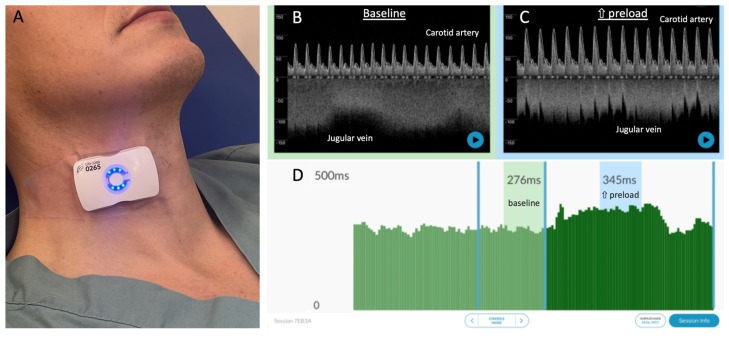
The wearable Doppler system and its graphical user interface. (A) The wireless, wearable Doppler transducer adhered to the neck of a healthy subject. (B) Representative resting baseline data from a healthy subject with common carotid and internal jugular spectrograms. (C) Representative data during increased preload showing carotid and jugular spectrograms; note the change in carotid and jugular morphologies consistent with increased preload and ccFT. (D) Per-beat ccFT calculations showing windows at baseline (average ccFT 276 ms) and during increased preload (average ccFT 345 ms).
